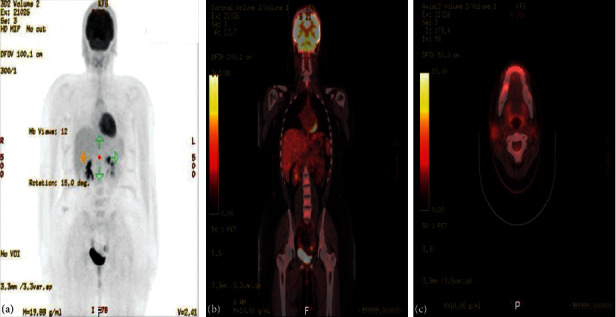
The PET scan in November 2017. (a)–(c) There is mild background level FDG avidity (SUVmax 2.2) in the region of the right parotid. This uptake is most likely postsurgical. Also, there is mild FDG avidity (SUVmax 1.7) within a few prominent (up to 11 mm short axis) right upper cervical (levels II-III) lymph nodes. The exact etiology remains indeterminate (i.e., whether could be malignant or reactive due to her recent surgery). Also, small volume lymph nodes (measuring up to 8 mm short axis) are present in the left upper cervical/level II region, with minimal FDG uptake, as this is a common site for reactive lymphadenopathy. On the other hand, there are additional small volume bilateral submental and submandibular lymph nodes (up to 6 mm short axis) with no definite FDG uptake. Uptake of the tracer elsewhere is within physiological limits.
